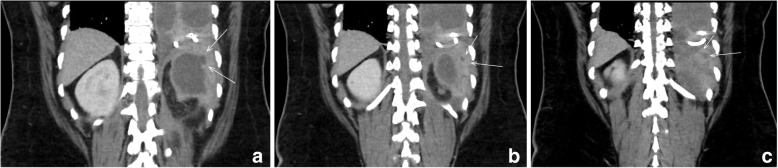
Computed Tomography results showing sequential coronal cross sections of the patient’s abdomen extending posteriorly with the white arrows indicating the posteriorly and superiorly extending tract from the renal cyst to the diaphragm. (Anterior to Posterior (Left to Right): a, b, and c)
